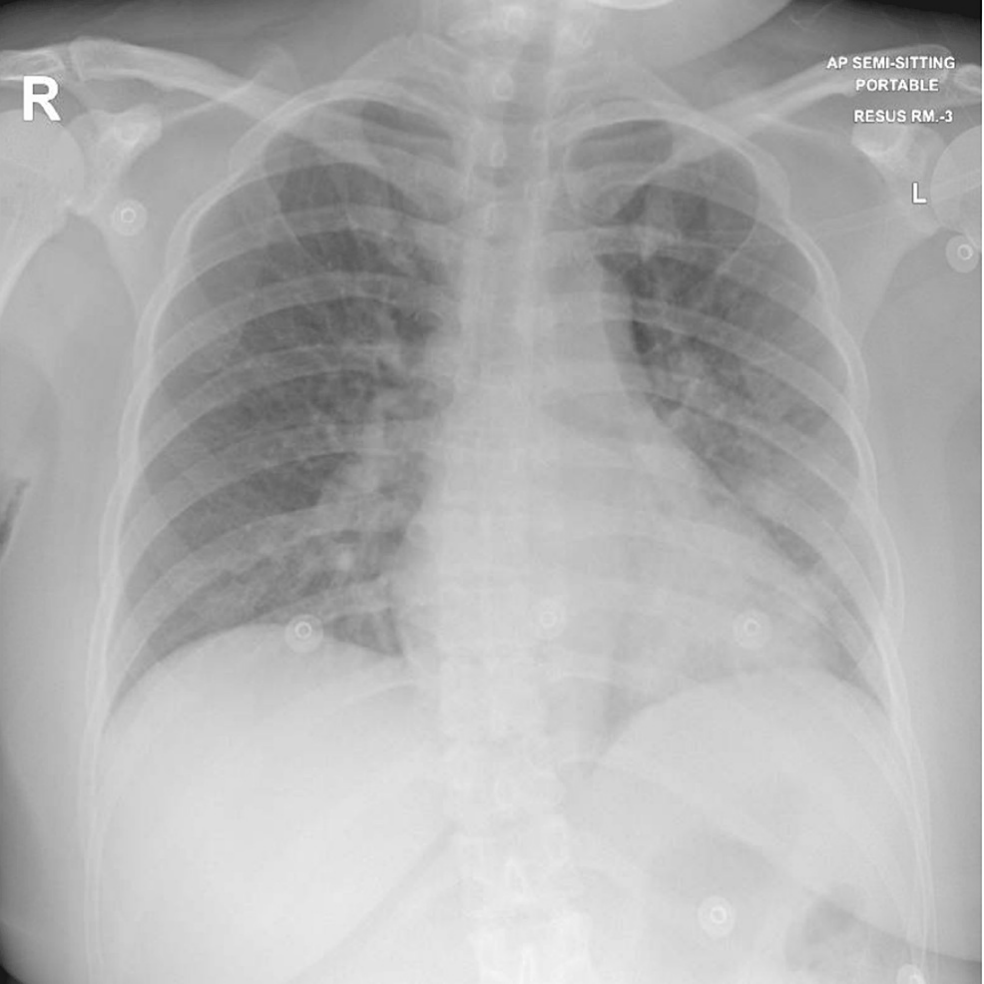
Initial chest X-ray showing cardiomegaly and non-homogenous opacities in mid and lower zones of the left lung
